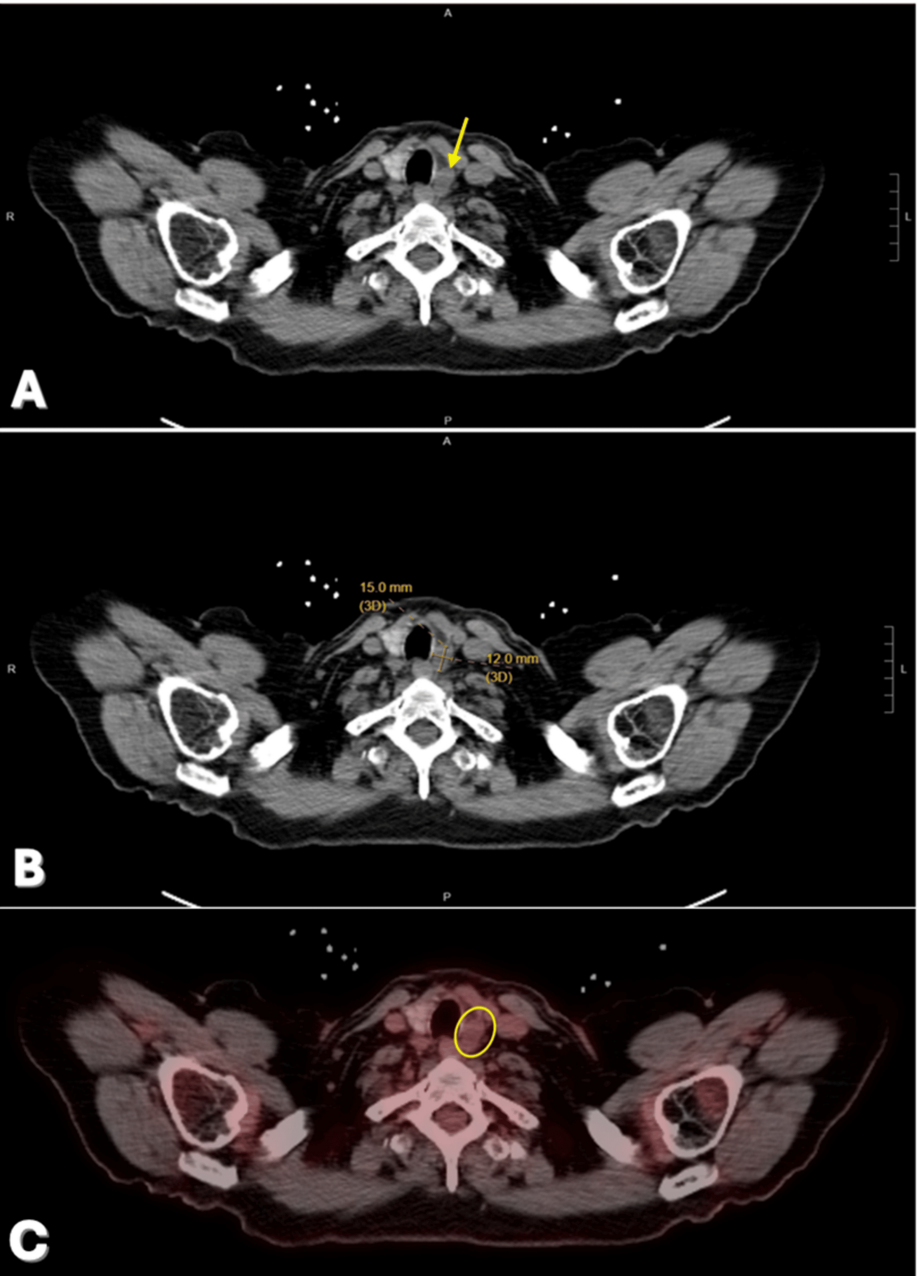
(A) A Chest CT scan of left Paratracheal Lesion. The lesion appeared adjacent to the left thyroid lobe (arrow). (B) Anterior position of the patient identifying a 1.5 × 1.2 cm left paratracheal lesion suggestive of nodal metastasis. (C) A positron emission tomography (PET) and computed tomography (CT) (PET/CT) scan of left Paratracheal Lesion demonstrated no FDG avidity (SUV max 1.2) (circle). A: Anterior, P: Posterior, L: Left, R: Right
